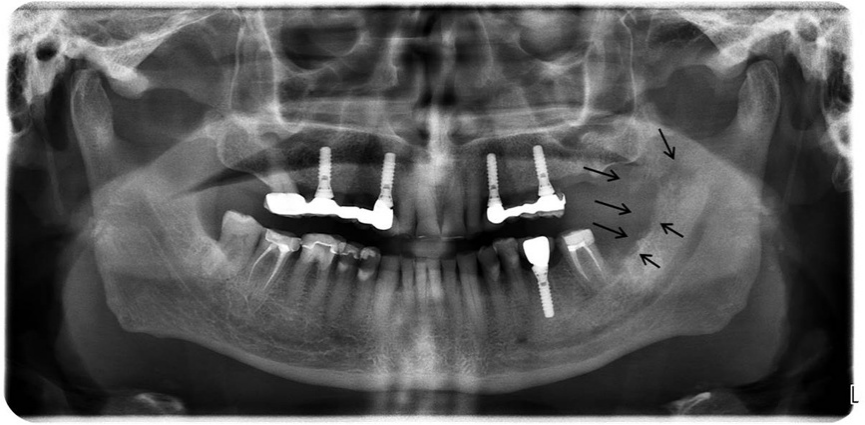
Panoramic radiograph taken 1.5 months later and showing severe erosion on the left site of the mandible.
